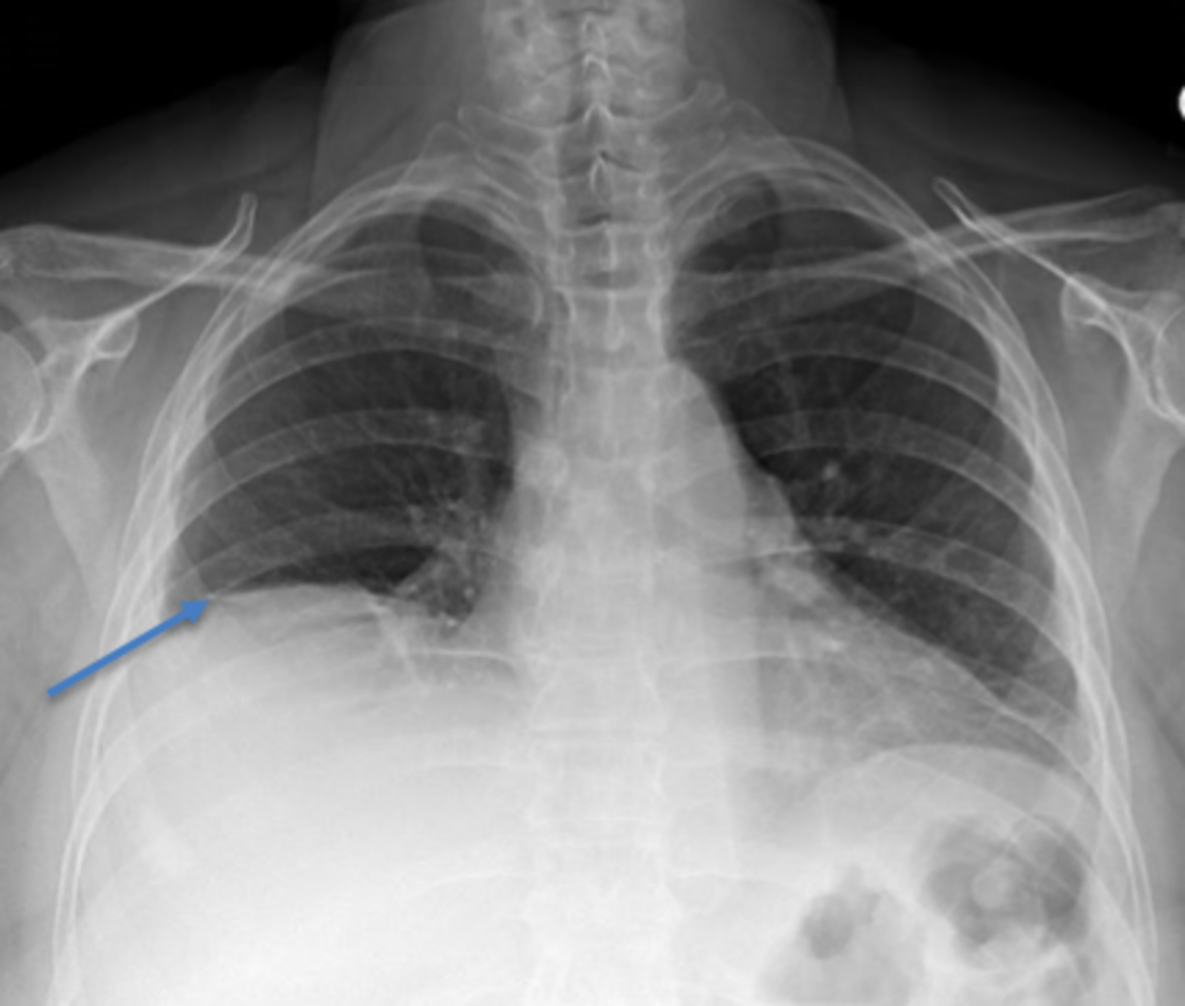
Chest X-ray showing low lung volume, elevated right hemidiaphragm, and normal pulmonary parenchyma.
